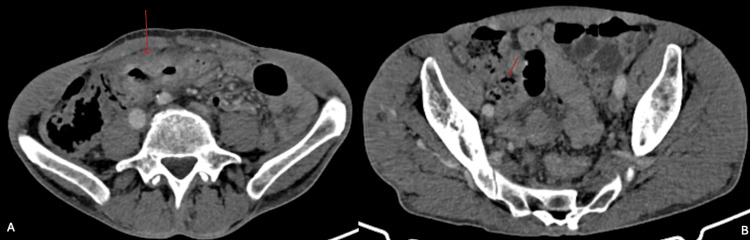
Abdominal CT - Case 2 Case 2. A: Tumor in the transverse colon. B: Appendix partially visualized. The CT reported circumferential parietal thickening in the transverse colon, close to the hepatic flexure, with luminal reduction of the colon. No alterations were reported in the appendix.
